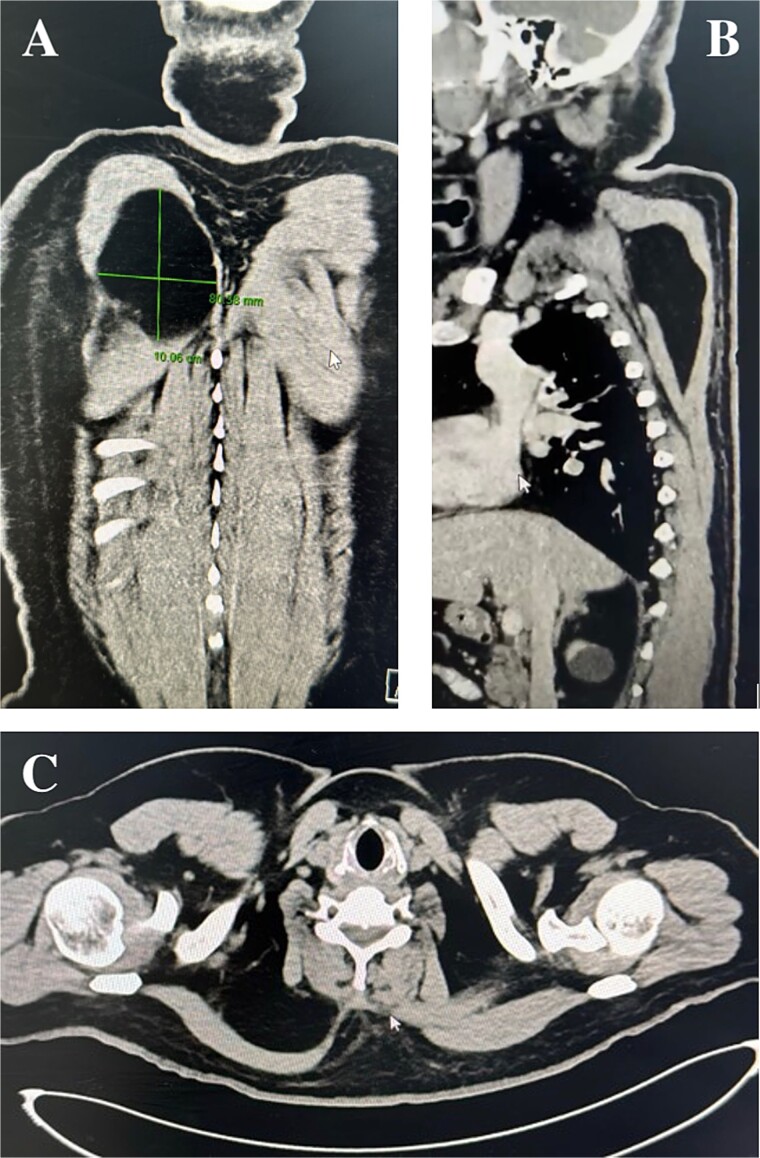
Computed tomography scan: Coronal, sagittal, and axial planes of the lipoma.
